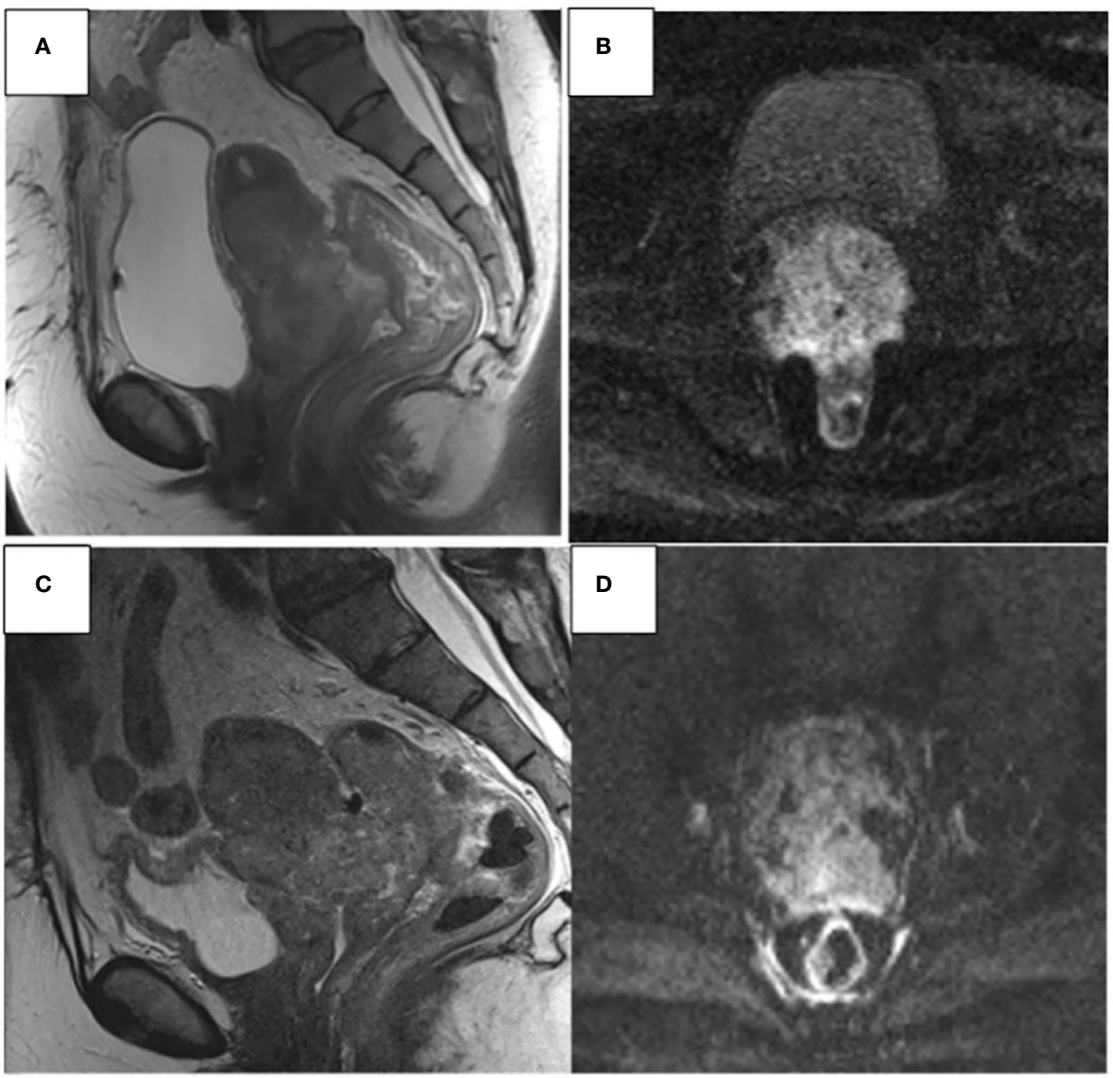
67- year-old woman. Sagittal T2W and axial DWI MR images show an invasive CC (A, B). Progression disease (C, D) CC infiltrates the uterine body, lower 1/3 of the vaginal canal, mesorectum, anterior wall of the rectum, and posterior wall of the bladder.
